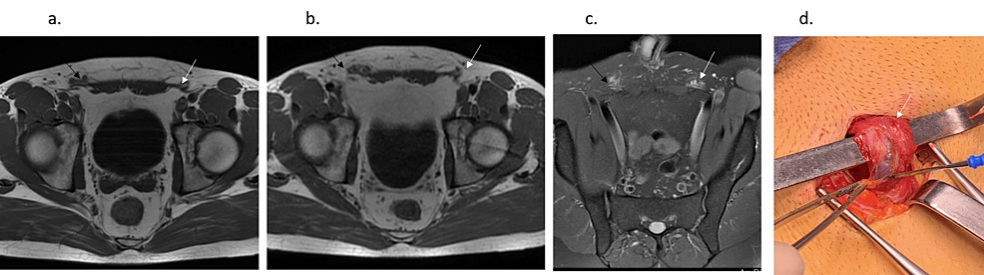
A 33-year-old man, professional soccer player (Second league) with bilateral pain. Axial T1 in Abdominal Wall (AW)-related Chronic Groin Pain (CGP) (a) and Axial oblique Proton Density weighted with Fat Saturation (PDFS) in AW (c), with bilateral bulging (bilateral fatty overload): grade 3 in right inguinal orifice (black arrow) and grade 2 in left inguinal orifice (white arrow) in these static MRI sequences. This AW bilateral lesion becomes grade 4 in the right inguinal orifice and grade 3 in the left inguinal orifice in axial T1 VM (b) (same patient, same level). Left AW exposed in AW-related CGP with black arrow = spermatic cord (covered by external oblique aponeurosis).
